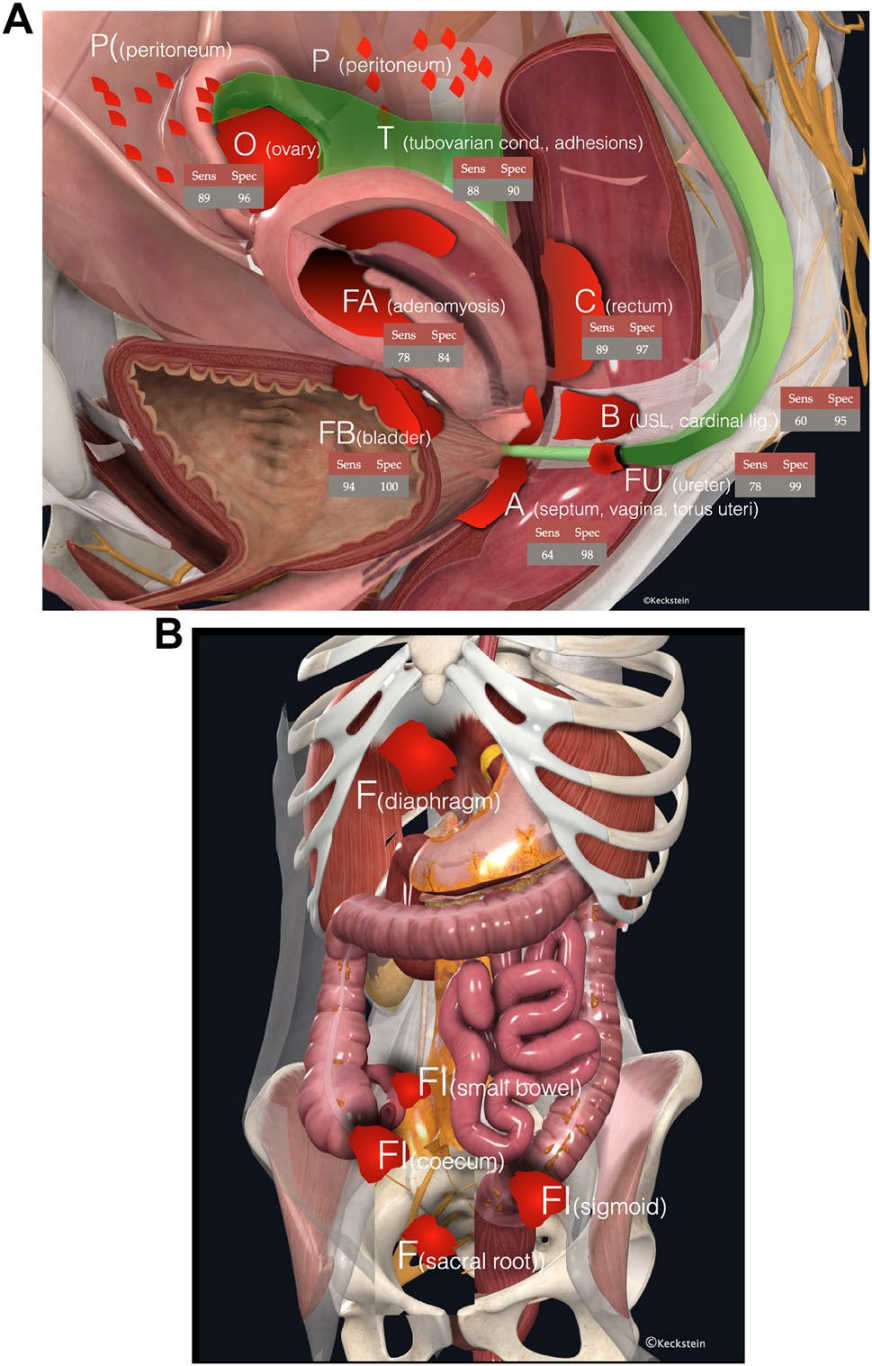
A The different compartments (P, O, T, A, B, C, FA, FB, and FU) in the pelvis subdivided according to the #Enzian classification, including data on the accuracy of TVS in the diagnosis of endometriosis. (sens. = sensitivity, spec. = specificity). Data derived from meta-analyses and individual studies show a large variance, which can be explained by different investigators and, in some cases, not yet standardized examination techniques. This is confirmed by the fact that TVS has a very high specificity in most compartments. The data are given in %. References: O [4, 6, 19], T [6, 19], A [5, 19, 36], B [5, 19, 36], C [19, 24, 35, 55], FA [39], and FU [19]. B Visualization of the other F compartments (except FA, FB, and FU) according to the #Enzian classification. To date, there are insufficient valid data on the accuracy of sonographic diagnostics in these compartments
